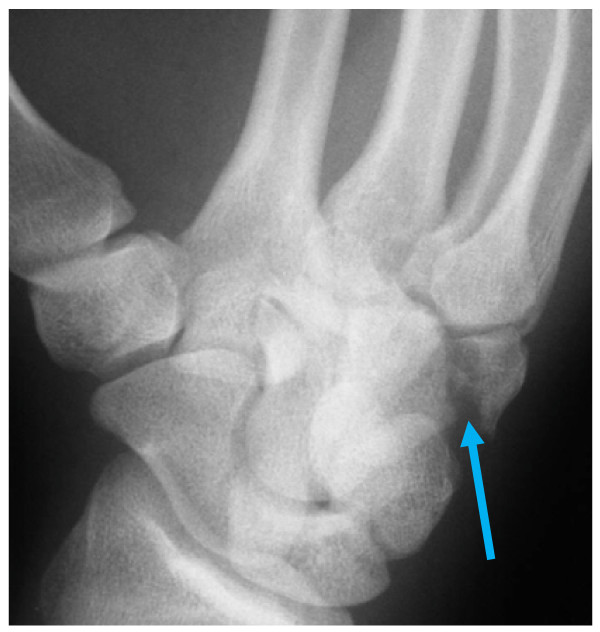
Pronated oblique 30° x-ray view. Blue arrow shows fracture site.
